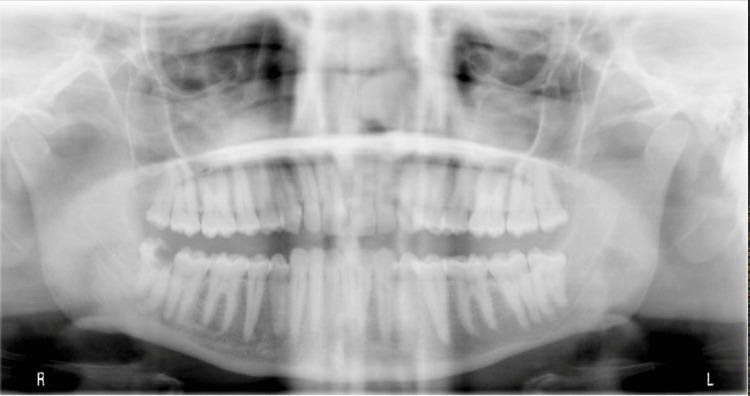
A pre-extraction orthopantomogram of all wisdom teeth
